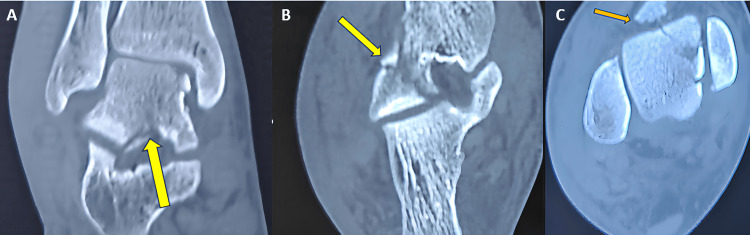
CT images of the right ankle. The images show (A) coronal view showing talus fracture extension into subtalar joint (yellow arrow), (B) axial view showing talus fracture extension into subtalar joint (yellow arrow), and (C) axial view showing talus fracture extension and fractured osteochondral fragment (yellow arrow).
